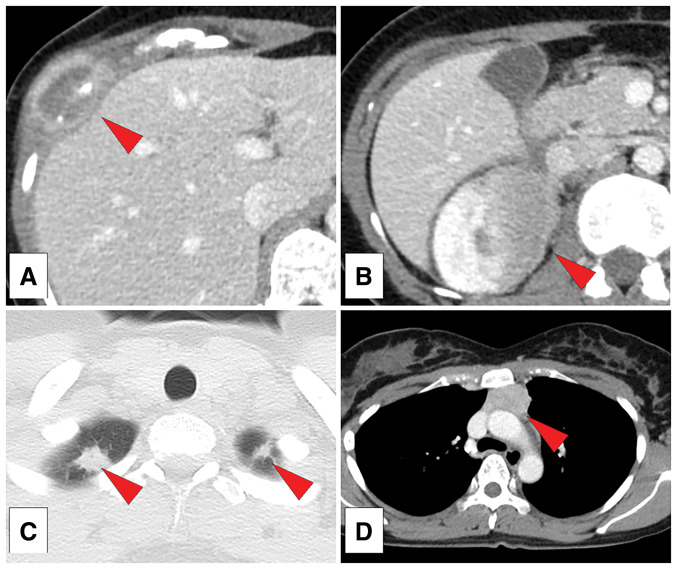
Computed tomography (CT) with contrast. (A) A 30-mm-wide mass with osteolytic changes in the right eighth rib. (B) A 42-mm-wide right renal mass. (C) Bilateral lung nodules with diameters of 13 mm and 6 mm. (D) A 34-mm-wide anterior mediastinal mass.
